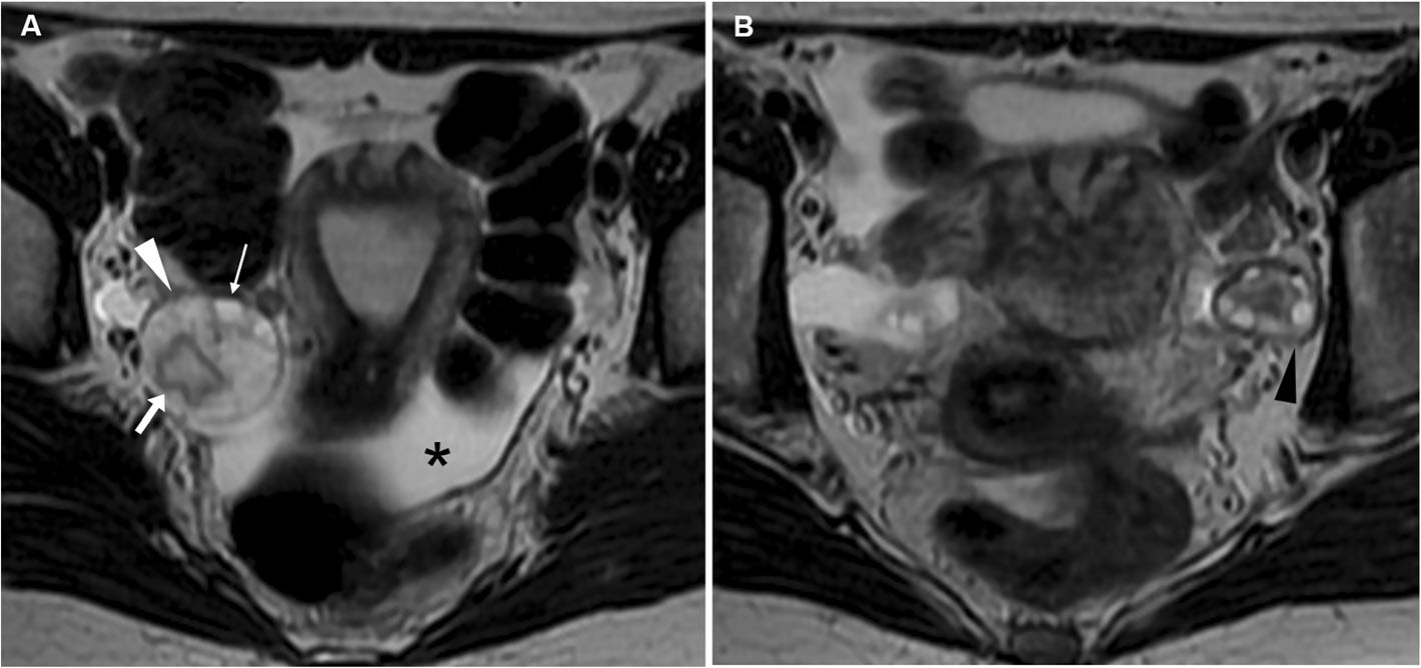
Unilateral massive ovarian oedema in a 23-year-old woman with recurrent self-limiting episodes of acute pelvic pain in the last year. Oblique coronal T2-weighted image (a) shows enlargement (4 cm diameter) of the right ovary (arrowhead) characterised by oedematous hyperintense stroma; follicles (thin arrow) and corpus luteum (arrow) are displaced at the periphery of the cortex. Moderate peritoneal effusion (asterisk) coexists. Oblique coronal T2-weighted image (b) demonstrates the normal-appearing left ovary (black arrowhead)
